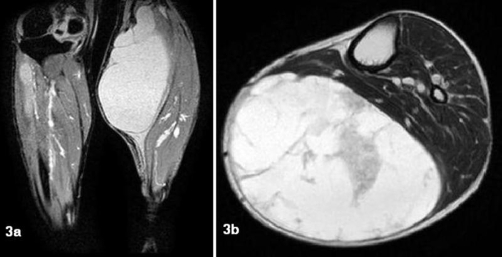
MRI showing a cystic lesion in the posteromedial aspect of the knee and calf.
